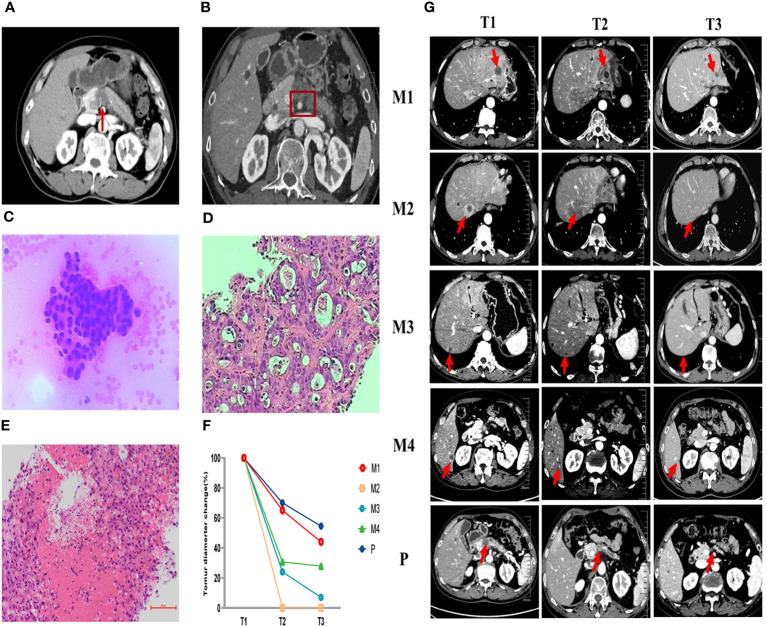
Pathological and imaging evaluations during the first-line (AG) and second-line (SOXPR) treatment. (A, B) A 2.2cm × 2cm mass at the neck of the pancreas was detected with superior mesenteric artery (SMA) invasion less than 180° on abdominal CT at baseline. (C) Cancer cells were observed in pancreas biopsy (×200) at T0. (D) Ultrasound guided biopsy of the hepatic mass (M2) was concordant with liver metastasis of pancreatic ductal adenocarcinoma (×200) at T1. (E) Pathology of M2 showed inflammatory cells and no residual tumor cells (×200) at T2. (F, G) Abdominal CT during second-line (SOXPR) treatment and the tumor diameter variation. T0, The baseline prior to first-line therapy; T1, The baseline prior to second-line therapy; T2, Obvious relief of TEN symptoms; T3,: Three additional cycles of SOX to end; M1-M4, 4 hepatic metastases; P, primary pancreatic cancer locus.
